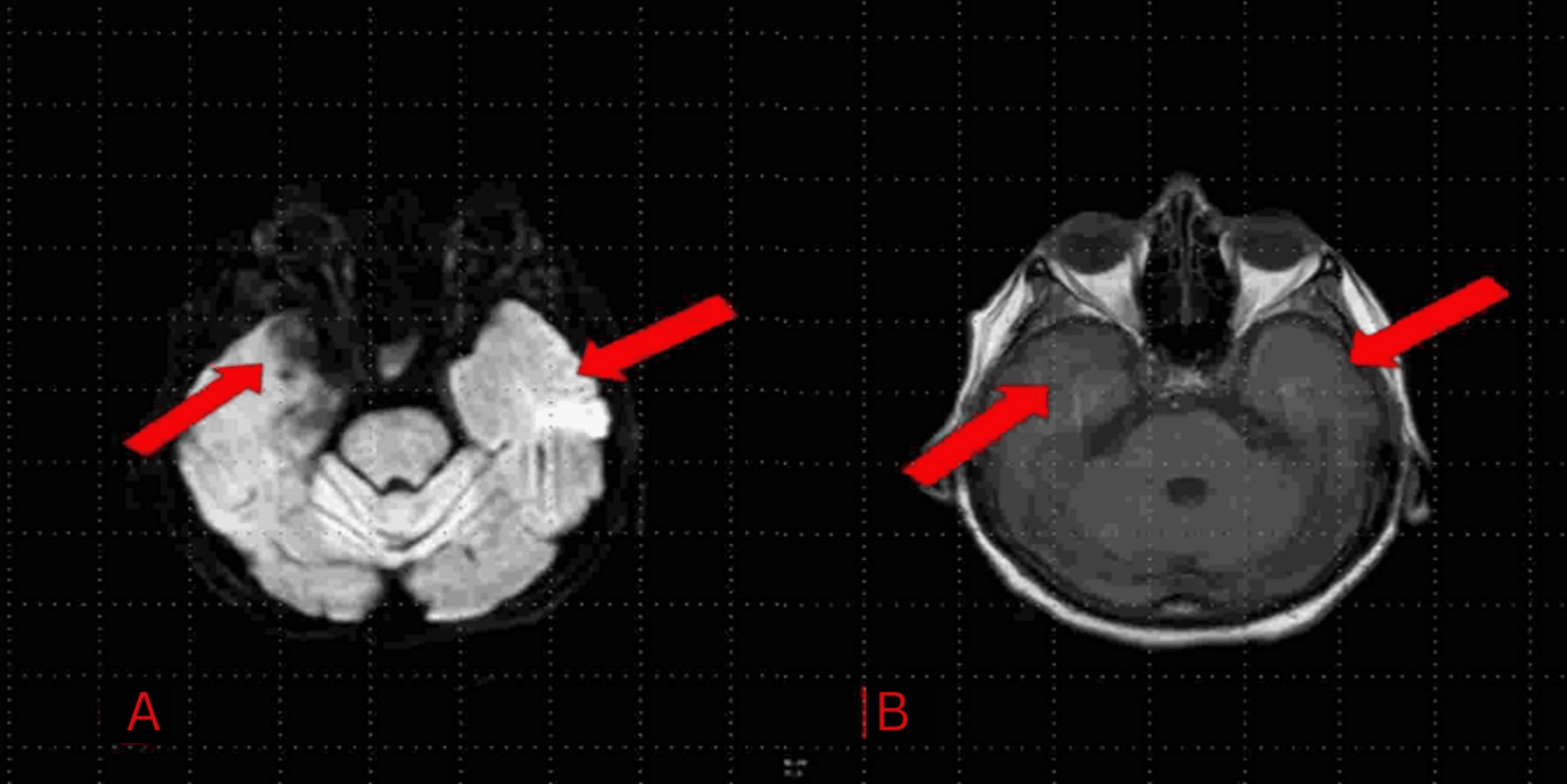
Focal subacute to chronic blood products in the right temporal lobe (A, B) consistent with inflammation and necrosis due to herpes simplex virus type 1 encephalitis (red arrows). The MRI was taken on day 18 to evaluate for improvements or worsening of the initial findings of the MRI on day 6 after treatment with acyclovir and ganciclovir.
